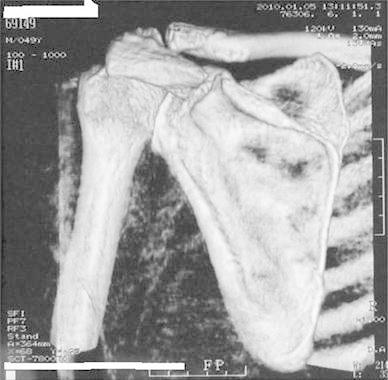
Frontal computed tomography (three dimensional)
